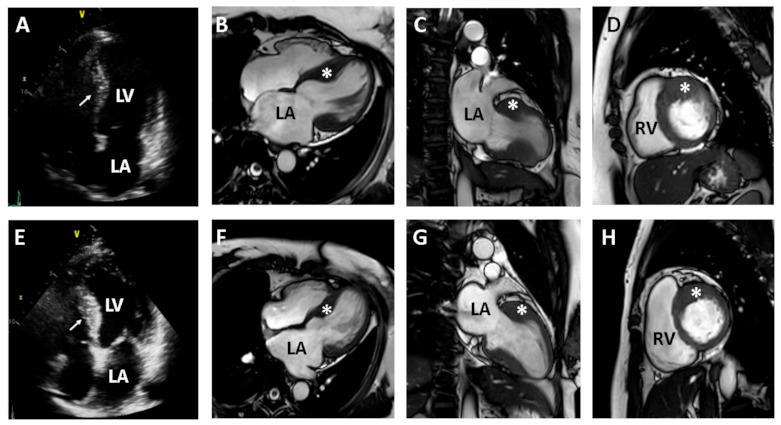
Two-dimensional echocardiography and contrast-enhanced cardiac magnetic resonance (CMR) images from the proband’s daughters with hypertrophic cardiomyopathy (HCM) (second daughter in upper panel and fourth daughter in lower panel). Two-dimensional echocardiography reveals the hypertrophy of the ventricular septum in each daughter ((A) in second daughter and (E) in fourth daughter). CMR images clearly demonstrate segmental hypertrophy (asterisk) confined to the ventricular septum, consistent with a diagnosis of HCM ((B–D) in second daughter and (F–H) in fourth daughter). LA, left atrium; RA, right atrium; LV, left ventricle; RV, right ventricle.
